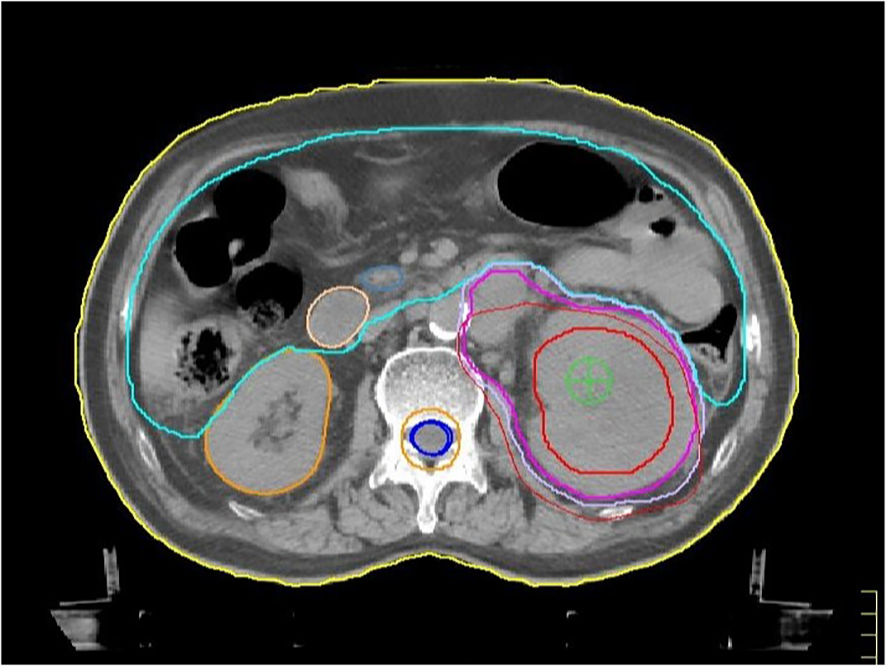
Delineation of radiation target volume. The axial enhanced CT image at the level of the largest diameter of the renal primary lesion, as planned using the Pinnacle system, shows the following: The Gross Tumor Volume (GTV) is outlined by the red solid line, indicating a 9.4×7.9 cm primary lesion in the left kidney. The Clinical Target Volume (CTV) is delineated by the purple solid line, representing the GTV expanded by 10 mm to include the high-risk retroperitoneal lymph node area. The prescribed dose is 60 Gy to the CTV, delivered in 30 fractions.
